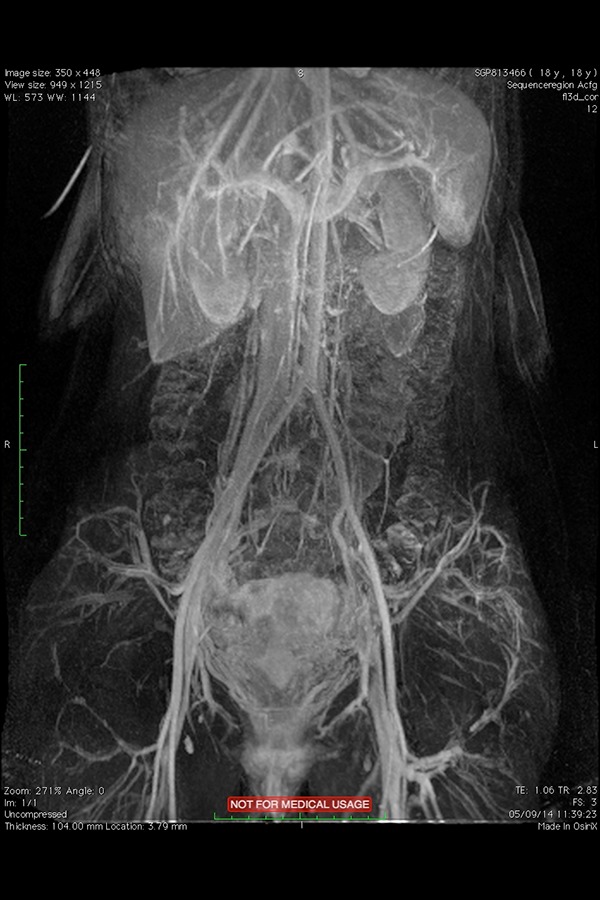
Subtracted maximum intensity projection coronal post contrast MRI showing dilated left pelvic collaterals.
